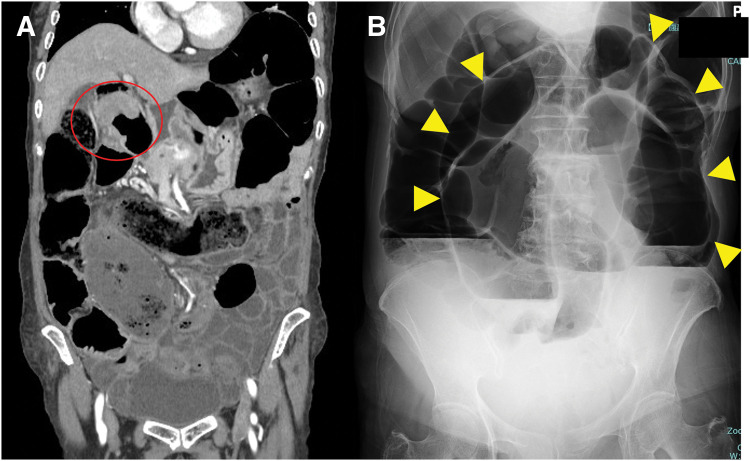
Preoperative imaging findings. (A) Contrast-enhanced CT (coronal view) showing a contrast-enhancing wall-thickening lesion located near the hepatic flexure of the transverse colon (red circle). No enlarged lymph nodes or apparent distant metastases were observed. (B) Abdominal radiograph demonstrating a markedly elongated sigmoid colon with torsion, consistent with sigmoid volvulus (yellow arrowheads).
